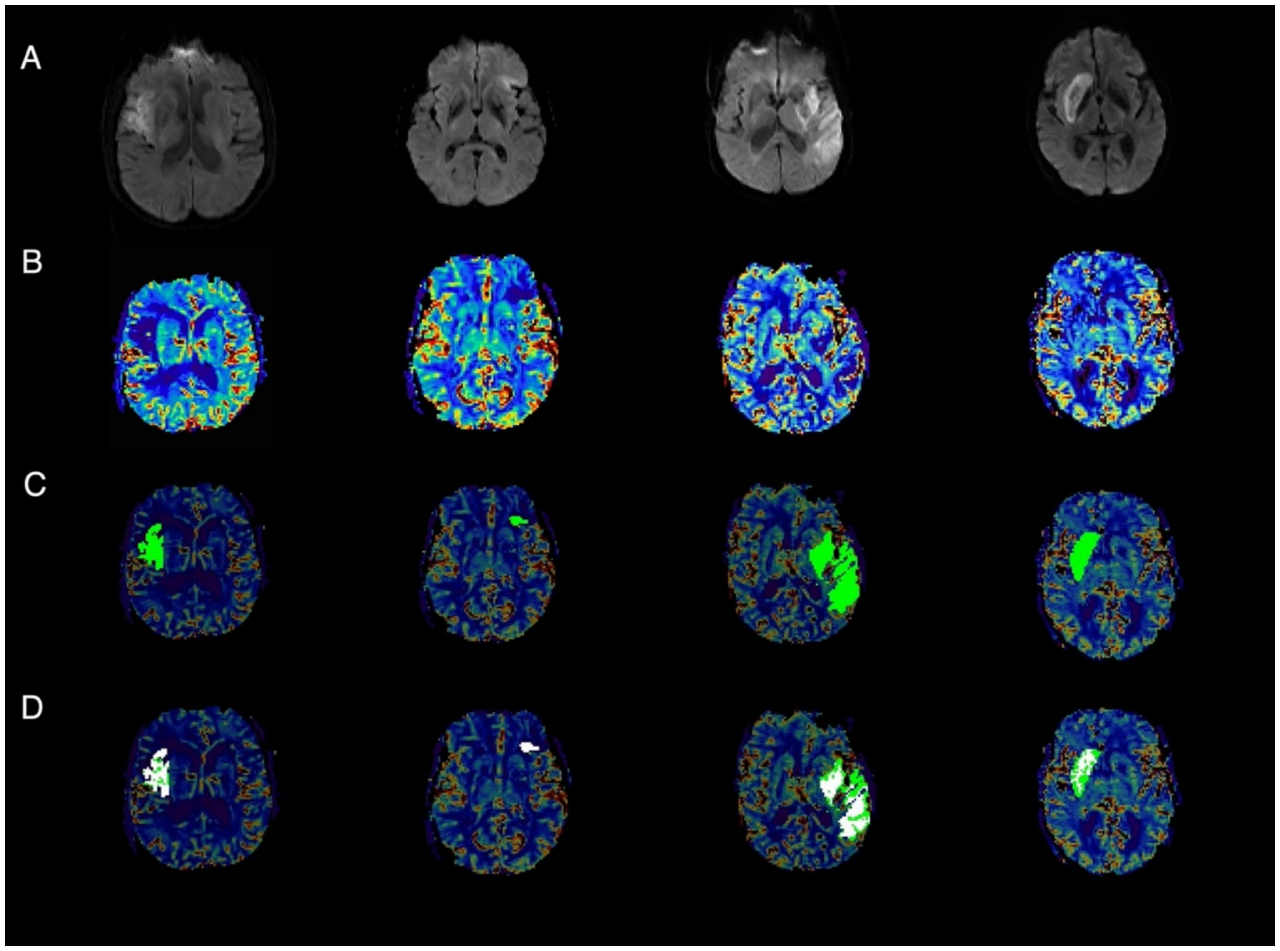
Representative cases of infarct microvascular impairment (IMR). (A) Post-EVT diffusion-weighted imaging (DWI); (B) Post-EVT relative cerebral blood volume (rCBV); (C) Post-EVT rCBV with overlaid infarct area (light green); (D) Post-EVT rCBV showing the infarct area (green) and regions of IMR (white).
